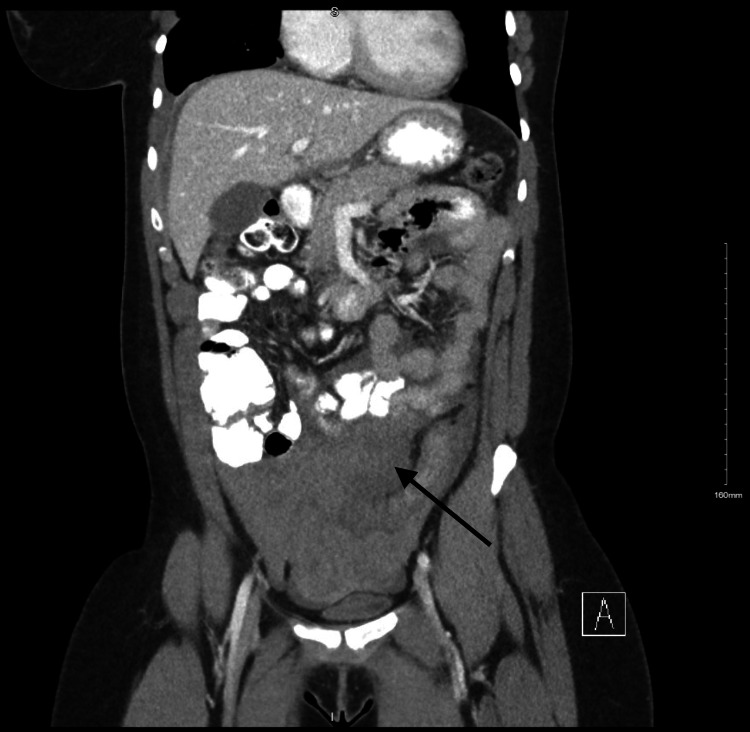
Computed tomography of the abdomen and pelvis (CT AP) with pelvic hematoma Coronal section of the CT AP performed on the day that the patient presented, showing a large volume of hemoperitoneum (arrow).
